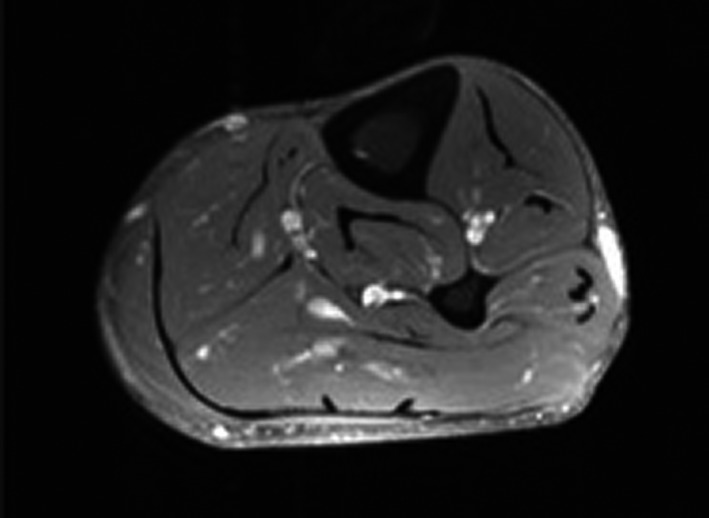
Axial T1‐weighted free spin magnetic resonance image of the left lower leg showing an avidly enhancing, ovoid‐shaped subcutaneous nodule. The nodule has a thin tail of enhancement extending posteriorly to the level of the surgical scar. The mass intimately abuts the superficial fascia of the peroneal musculature, without extension deep to the fascia.
